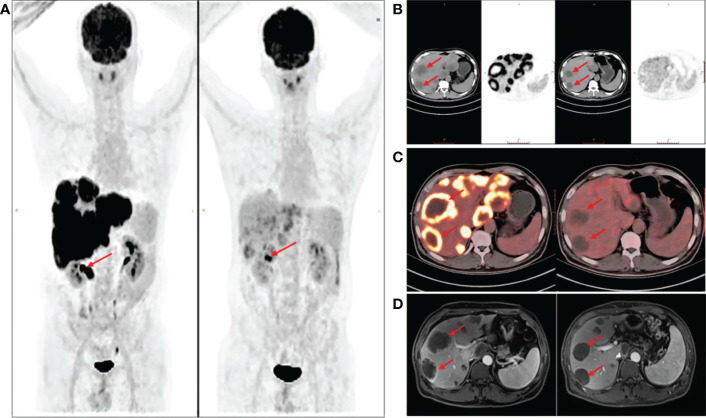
Drug-eluting beads transarterial chemoembolization (DEB-TACE), fluorodeoxyglucose-positron emission tomography/computed tomography (FDG PET/CT) assessment. Six weeks after the second DEB-TACE, FDG PET/CT (A) showed that the SUVmax of liver flexure and transverse colon lesions was decreased from 13.2 to 7.2. The SUVmax of some right lobe lesions was decreased from 14.0 to 5.3 (B, C) red arrow). Re-examination of contrast-enhanced abdominal MRI showed that the intrahepatic tumor volume was not significantly reduced, and the sum of target lesion diameters was less than 30% compared with the baseline level (D).
